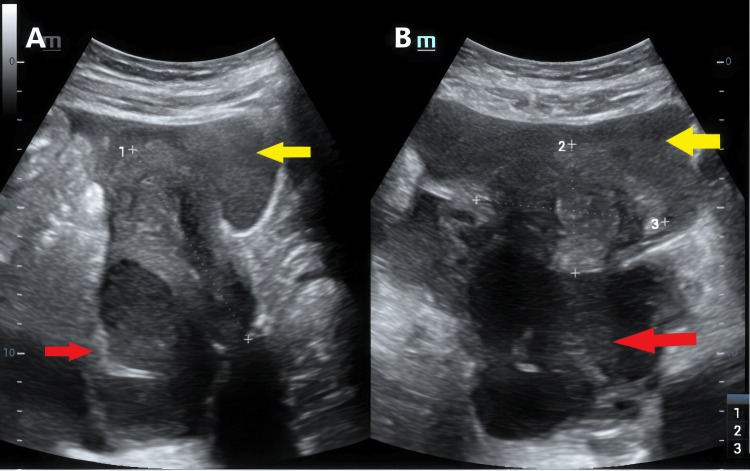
Ultrasound (sagittal and axial views) showing a retro-uterine, multi-loculated, thick-walled cystic structure with diffuse low-level internal echoes (red arrow) and intraperitoneal echoic free fluid (yellow arrow).
